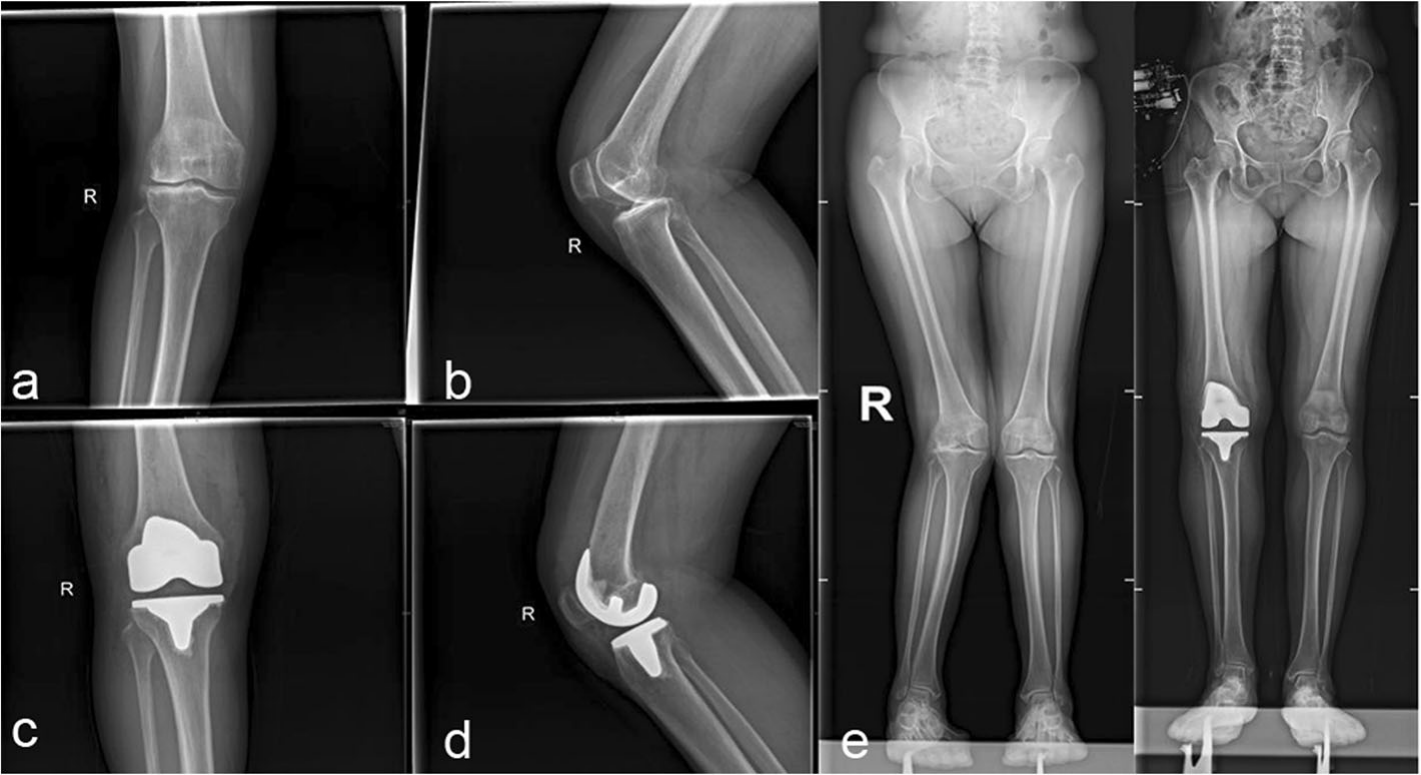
A 65-year-old female with osteoarthritis. a, b Preoperative anteroposterior and lateral X-ray showed that the narrow knee joint space and a large amount of hyperosteogeny. e Preoperative full length X-ray showed valgus deformity. c, d One year after operation, the anteroposterior and lateral X-ray showed that the prosthesis was in suitable position, and there was no light transmission zone at the interface between prosthesis and bone. f One year after the operation, full length X-ray showed correction of lower limb alignment
